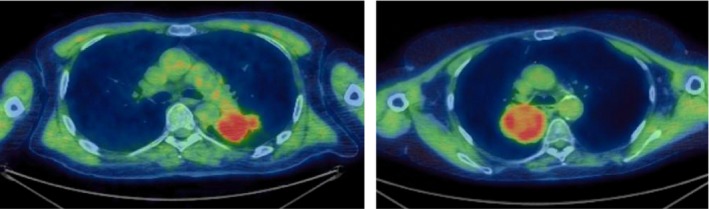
Two example FMISO PET/CT images 4 h p.i. (pre‐Buparlisib) on an SUV scale 0–3 for two different patients. [Color figure can be viewed at wileyonlinelibrary.com]
